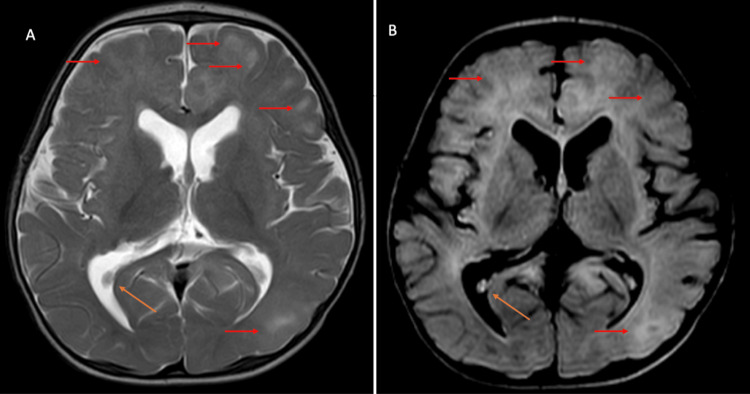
T2-weighted image (A) and fluid attenuation inverse recovery sequence (B) axial sections of the brain showing hyperintensities in the subcortical white matter (red arrows) and subependymal nodule (orange arrow)
